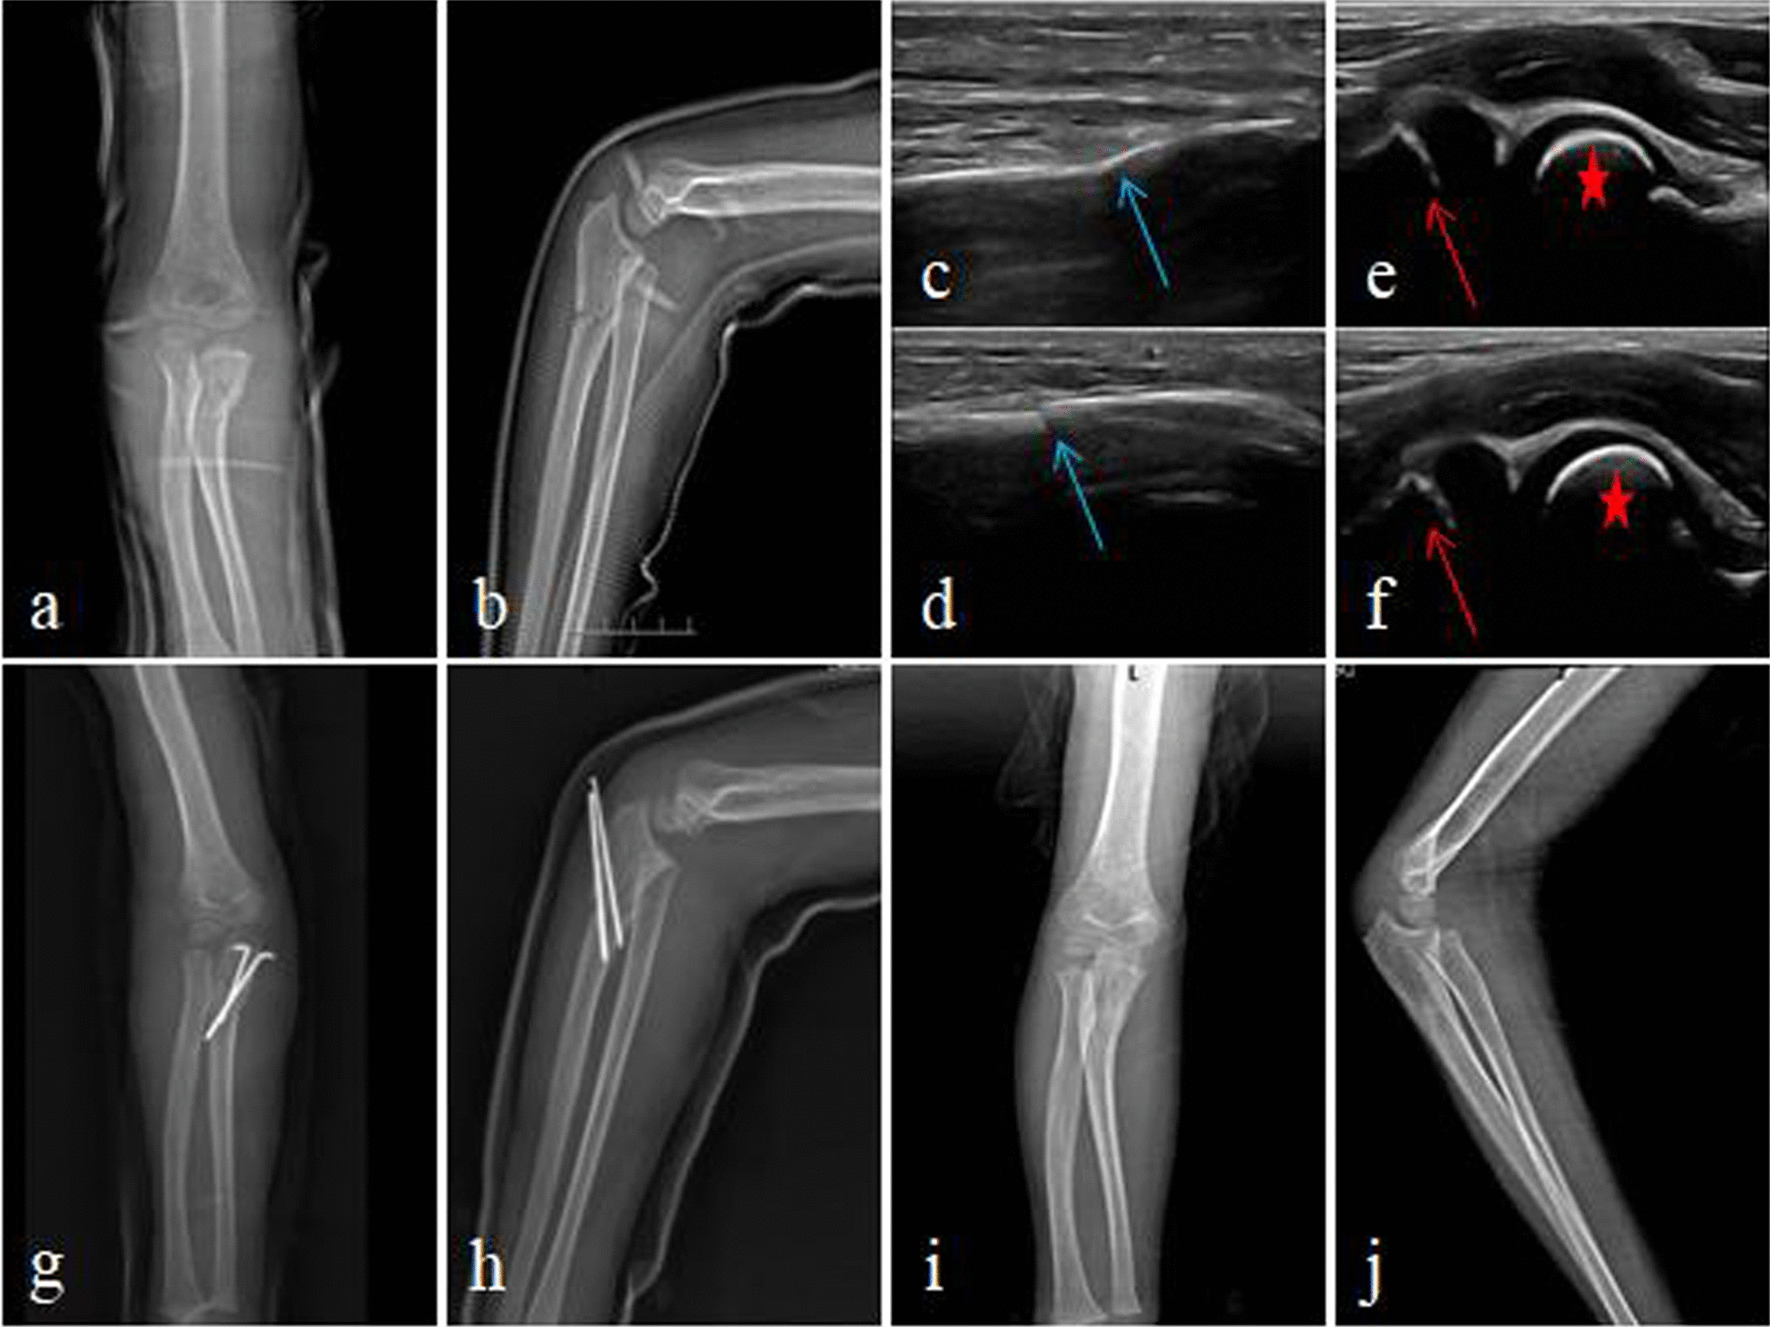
a, b Preoperative X-ray; c–f treatment of acute Monteggia fracture under ultrasonographic guidance (blue arrow—ulna fracture, red arrow—radial head, asterisk—capitulum humeri); g, h X-ray on the first day after surgery; i, j X-ray after removal of internal fixation
